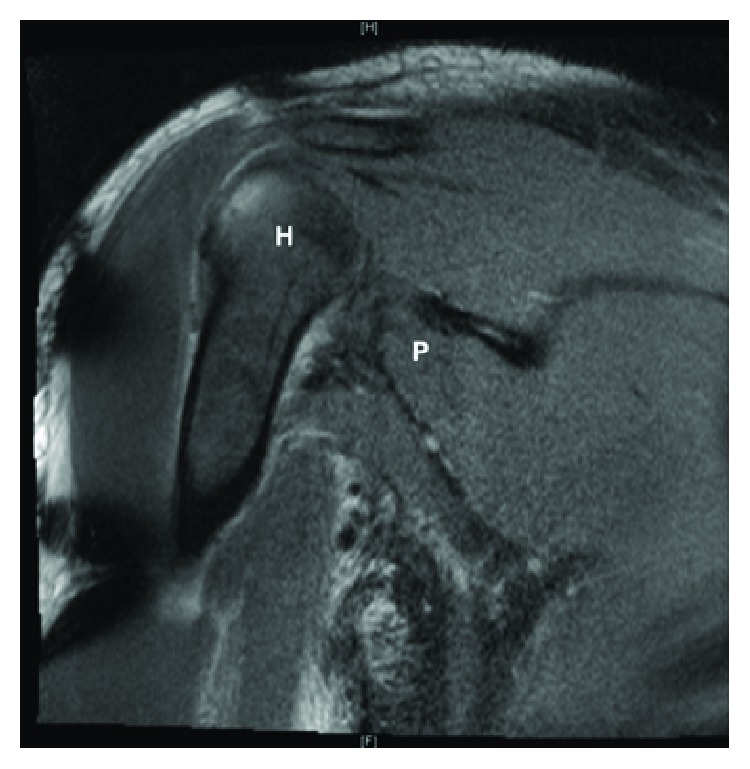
Coronal MRI image. H: humerus; P: retracted pectoralis major.
